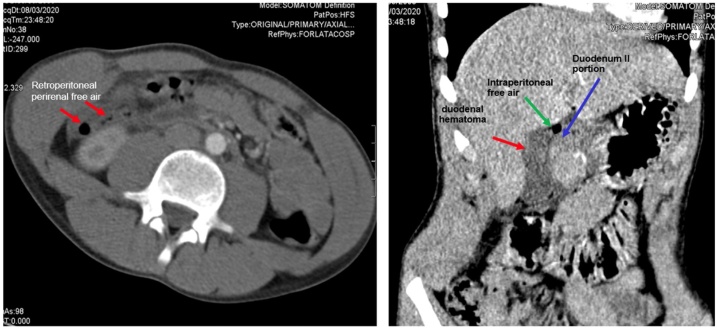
Abdominal CT scan showing retroperitoneal (a) and intrabdominal free air and a duodenal haematoma (b).
